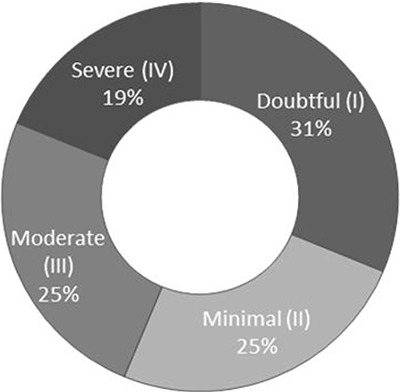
Knee osteoarthritis x-ray classification of the 16 volunteers of this study
